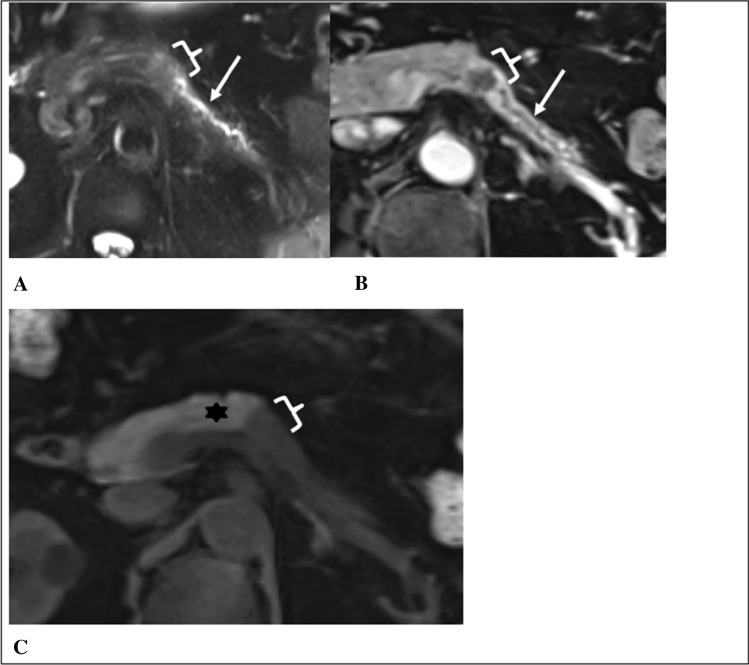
RADPEER 3 score on MRI—misinterpretation. 63-year old male who underwent an MRI because of an accidental finding of hepatomegaly on CT. Axial T2w fat saturated (A), axial T1w fat saturated venous phase (B), and axial T1w precontrast (C) images from an outside institution demonstrate pancreatic duct dilation (arrows) with abrupt terminating at a 25 mm hypoenhancing mass in the body (brackets). T1w precontrast images can be helpful for identifying the demarcation between normal hyperintense parenchyma (asterisks) and hypointense mass with atrophy. On initial report, this lesion was misinterpreted as a "likely benign cyst"; an example of misinterpretation with faulty reasoning and likely lack of knowledge (as a cyst would demonstrate T2 hyperintensity). Both expert readers identified the mass and secondary findings with high confidence. The patient was eventually diagnosed with metastatic pancreatic cancer 4.5 months later
